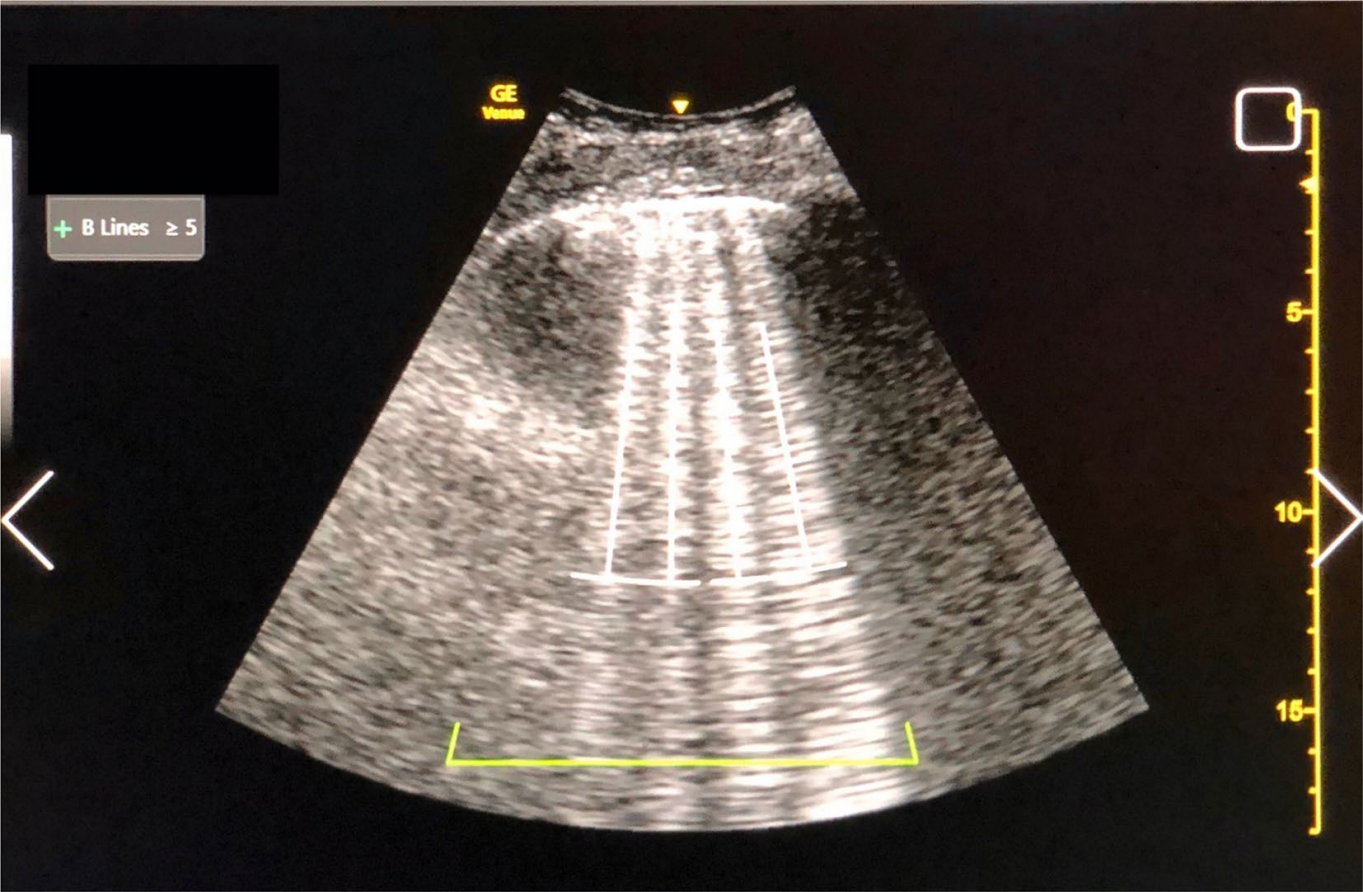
Lung ultrasound image of B-lines using machine AI for quantification. This image shows the zone analyzed (green line at bottom) and demonstrates ≥ 5 B-lines
